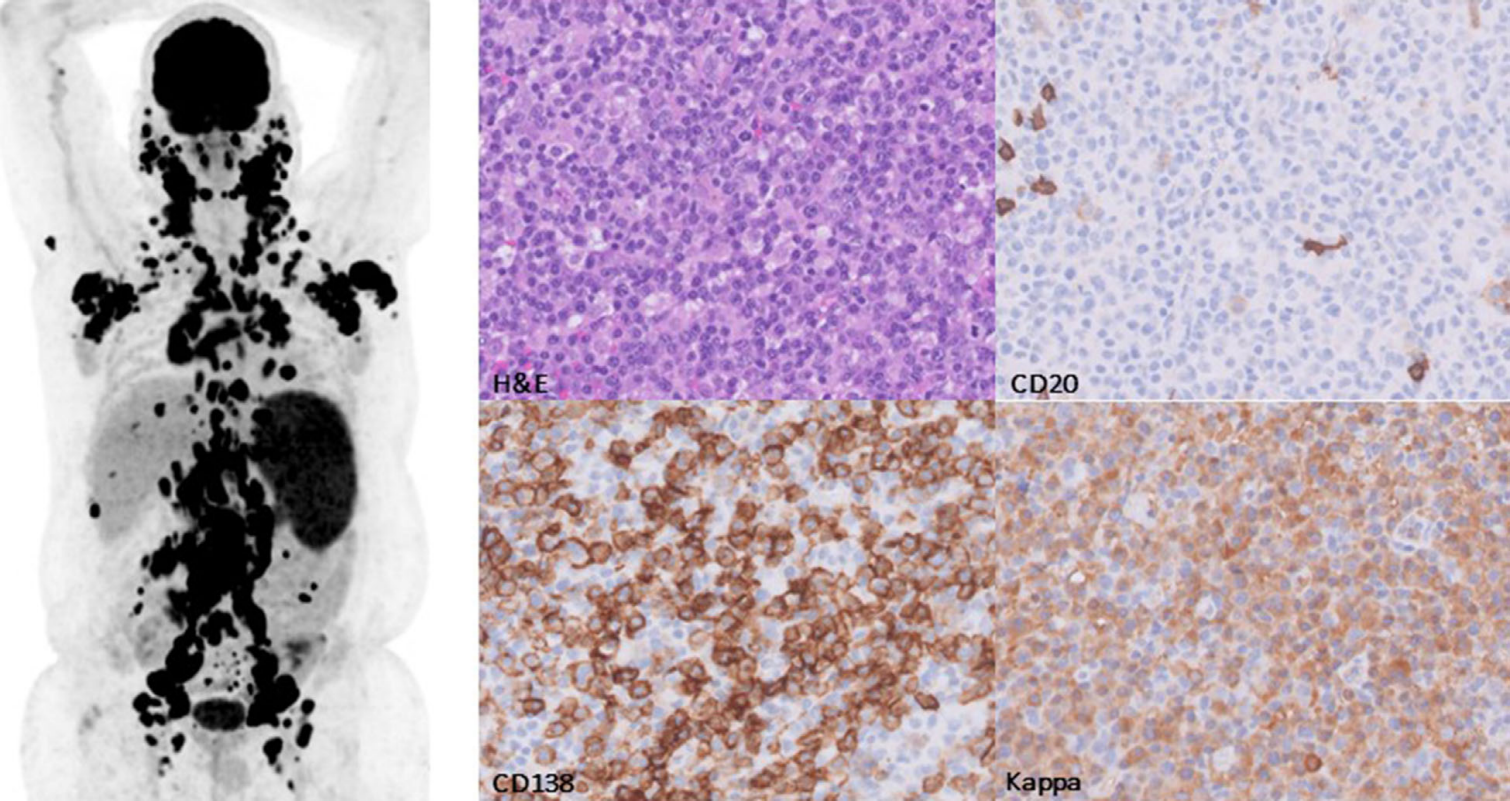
A 38‐year‐old male with a history of lupus nephritis and kidney transplantation 3 years ago, presented with fever, night sweats, and lymphadenopathy while being treated with tacrolimus, mycophenolate mofetil, and prednisone. Evaluation with PET and CT scan showed generalized lymphadenopathy and splenomegaly. (Left‐sided figure). Biopsy of an inguinal lymph node showed a monotypic plasma cell proliferation (CD138+, kappa+, CD20‐), as part of a monomorphic variant of post‐transplant lymphoproliferative disease (PTLD) (Right‐sided figure). The specific type was determined as plasmacytoma/multiple myeloma type PTLD. However, a diagnosis of PTLD type plasma cell‐rich marginal zone lymphoma was also possible, although no separate malignant lymphocytic B‐cell component could be demonstrated. No Epstein–Barr virus (EBV) positivity was found. Bone‐marrow showed a similar monomorphic PTLD with an increased amount of plasma cells. Laboratory testing showed only mild anemia and no kidney function decline or hypercalcemia. M‐protein was identified as IgA‐kappa, 24 g/L. There were no bone lesions. Cytogenetics of the bone marrow showed no abnormalities. A diagnosis of plasmacytoma/multiple myeloma type PTLD was made, and treatment was commenced with thalidomide, bortezomib, and dexamethasone.
